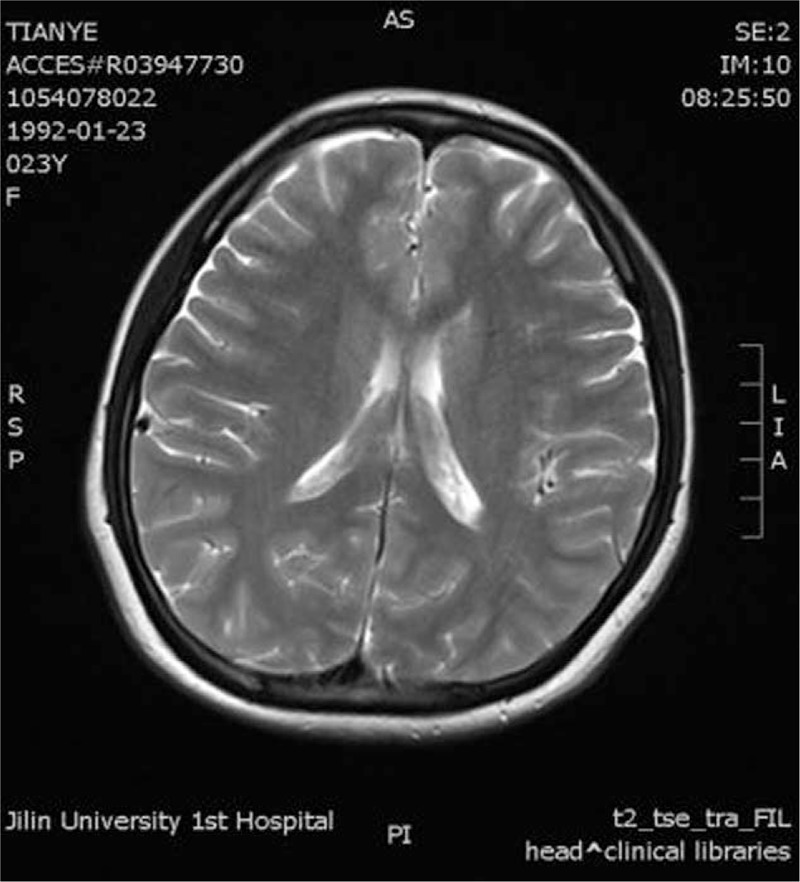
Craniocerebral magnetic resonance imaging showing no abnormality.
